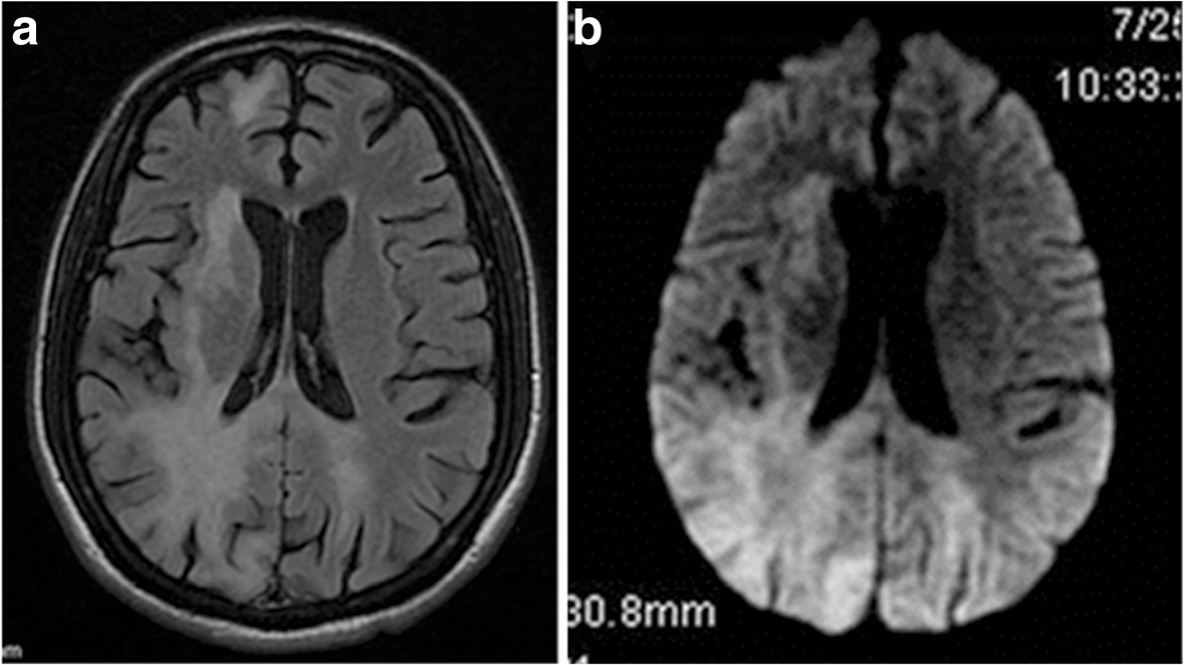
Axial T2-Flair MRI and axial T2-Flair diffuse-weighted image. (a) Axial T2-Flair MRI of the brain showing hyperintense signal intensity lesions bilaterally in the parieto-occipital white matter. (b) Axial T2-Flair diffuse-weighted image showing hyperintense lesions bilaterally in the parieto-occipital white matter.
